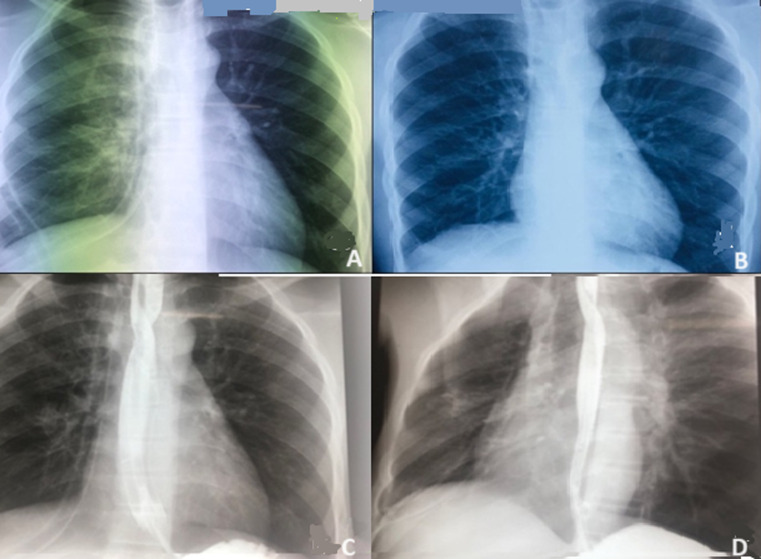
radiographies de contrôle post-opératoire: A) radiographie du thorax post-opératoire immédiat avec drains en place et petit pneumothorax droit apical; B) radiographie du thorax de contrôle à J45 post-opératoire; C, D) transit oeso-gastro-duodénal à la gastrografine de contrôle à J14 avec absence d´extravasation du produit de contraste
